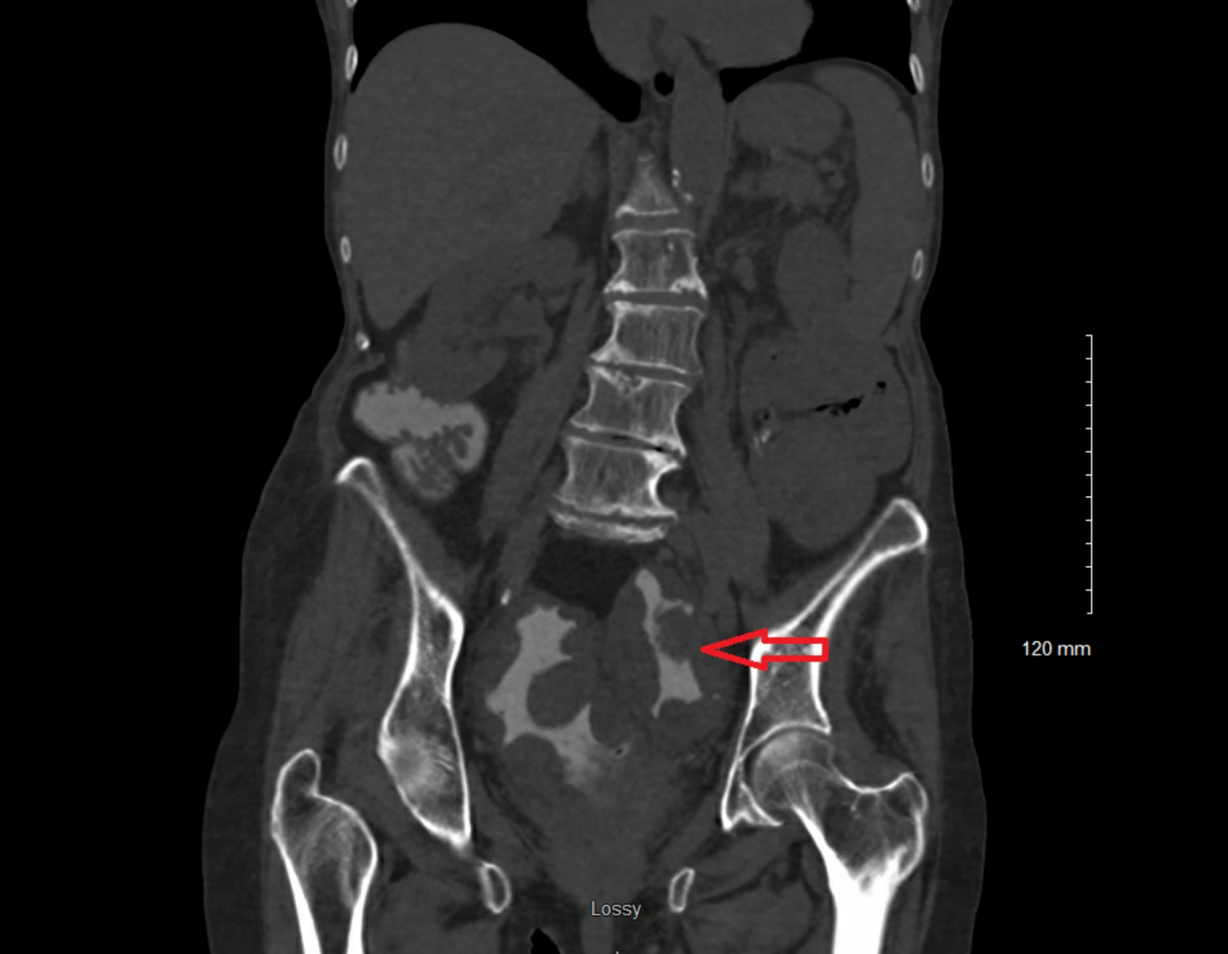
CT scan of the abdomen, arrow reveals severe thickening of the colon (red arrows).
